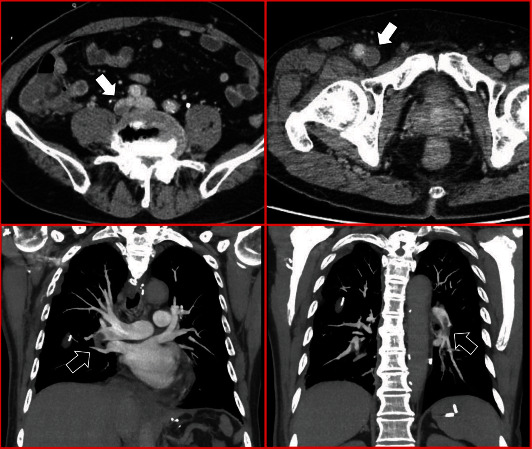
Contrast computed tomography imaging. White arrows indicate the region from the right iliac to the common femoral vein that was occluded by deep vein thrombosis, whereas black arrows indicate the bilateral pulmonary artery thromboembolism.
